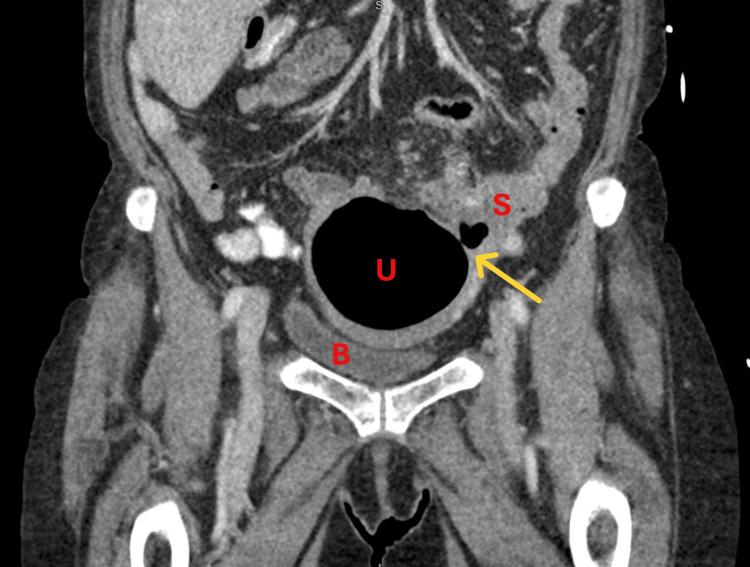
Contrast-enhanced computed tomography (CT) scan of the pelvis, coronal view. The fistulous tract (yellow arrow) is shown between the uterus (U) and sigmoid colon (S). Bladder (B) is labeled for reference.
